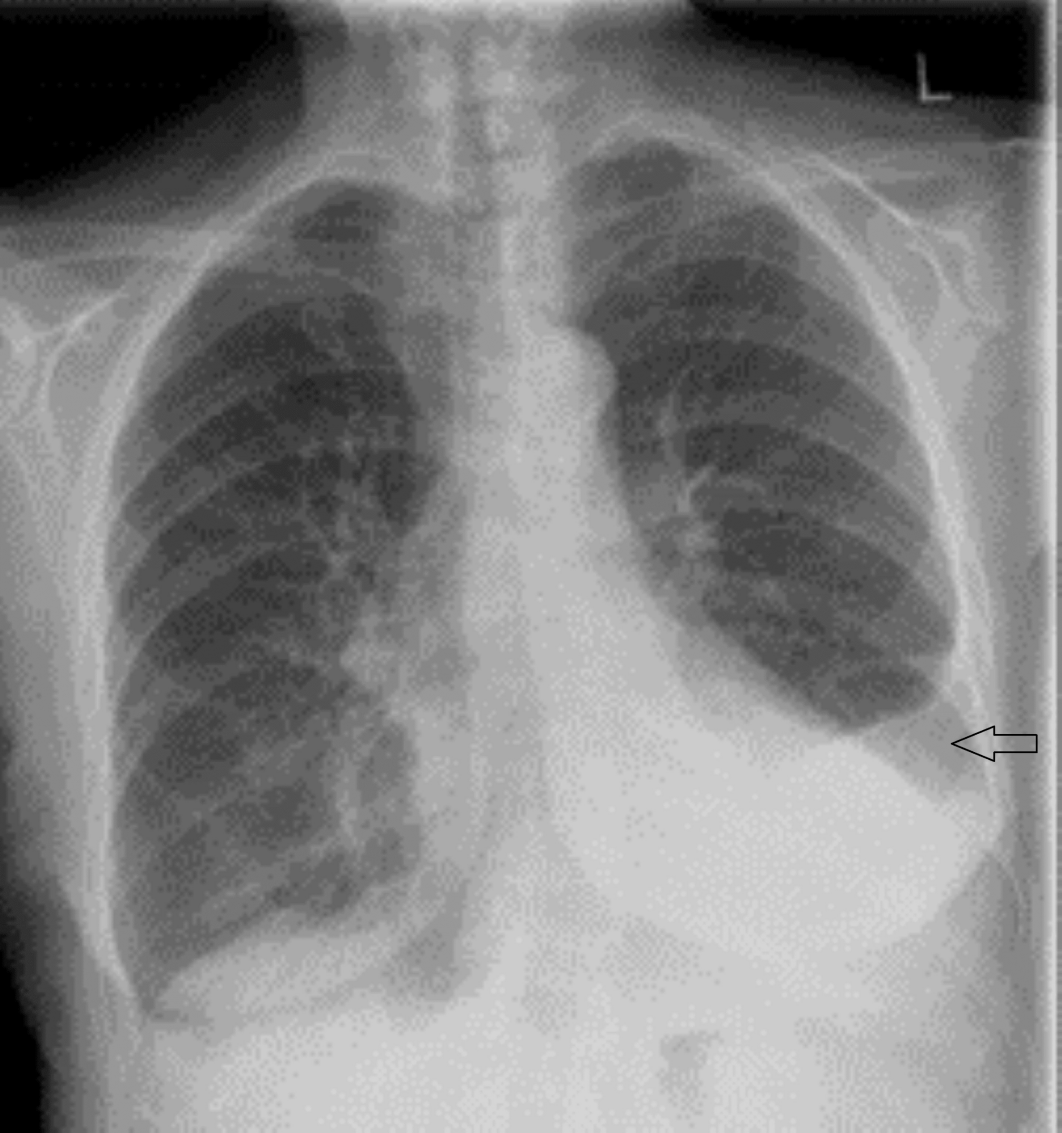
Chest X-ray (posteroanterior view) showing a moderate-sized left-sided pleural effusion.
